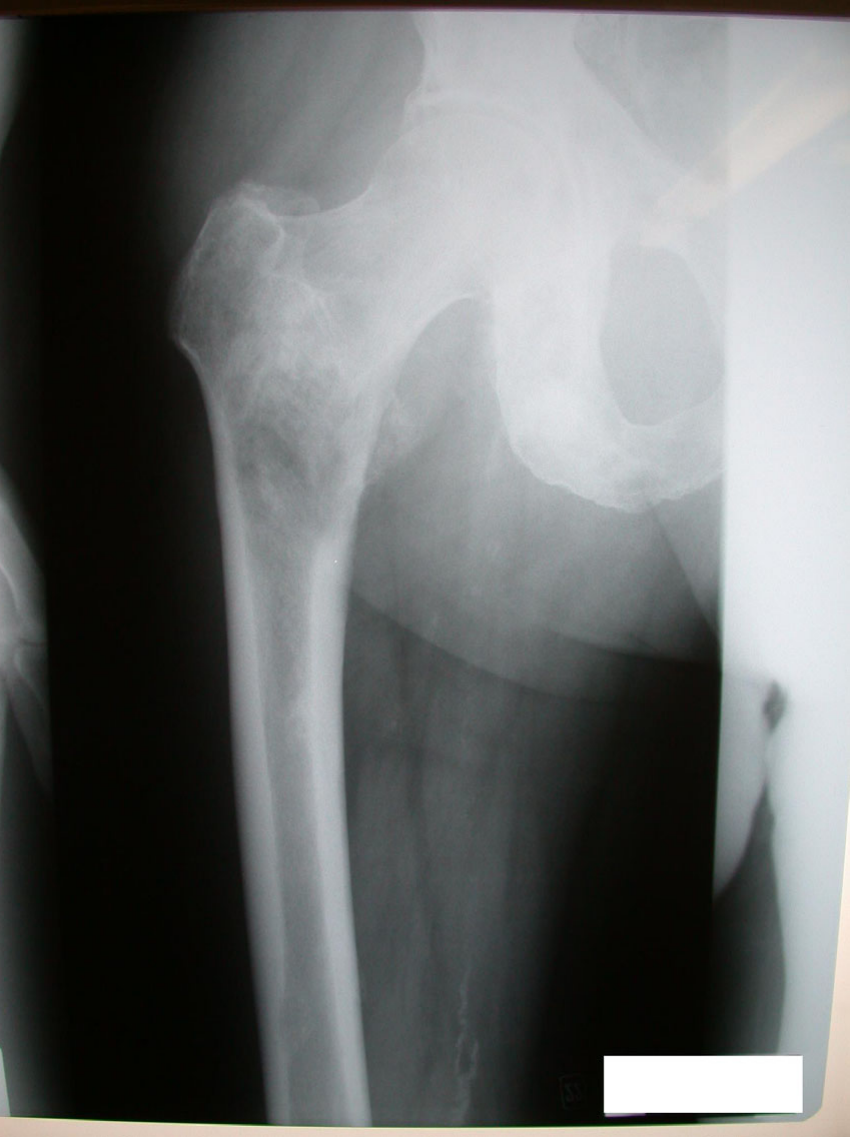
Anteroposterior (AP) view radiograph of the left femur demonstrating the large lytic metastasis in the subtronchanteric area.
